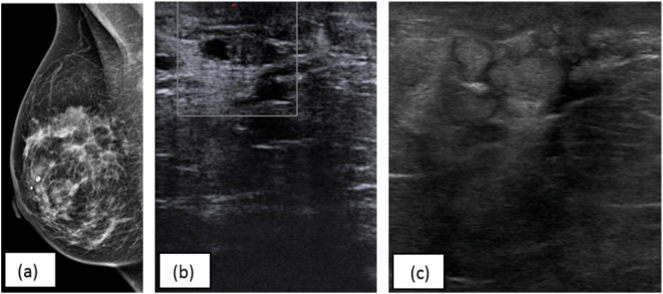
(a) Mammography suggests a retroareolar cyst (b) Ultrasound before ductoscopy: dilated retroareolar ducts (c) Ultrasound after ductoscopy: duct ectasia, not suspicious for an infection or malignancy.
